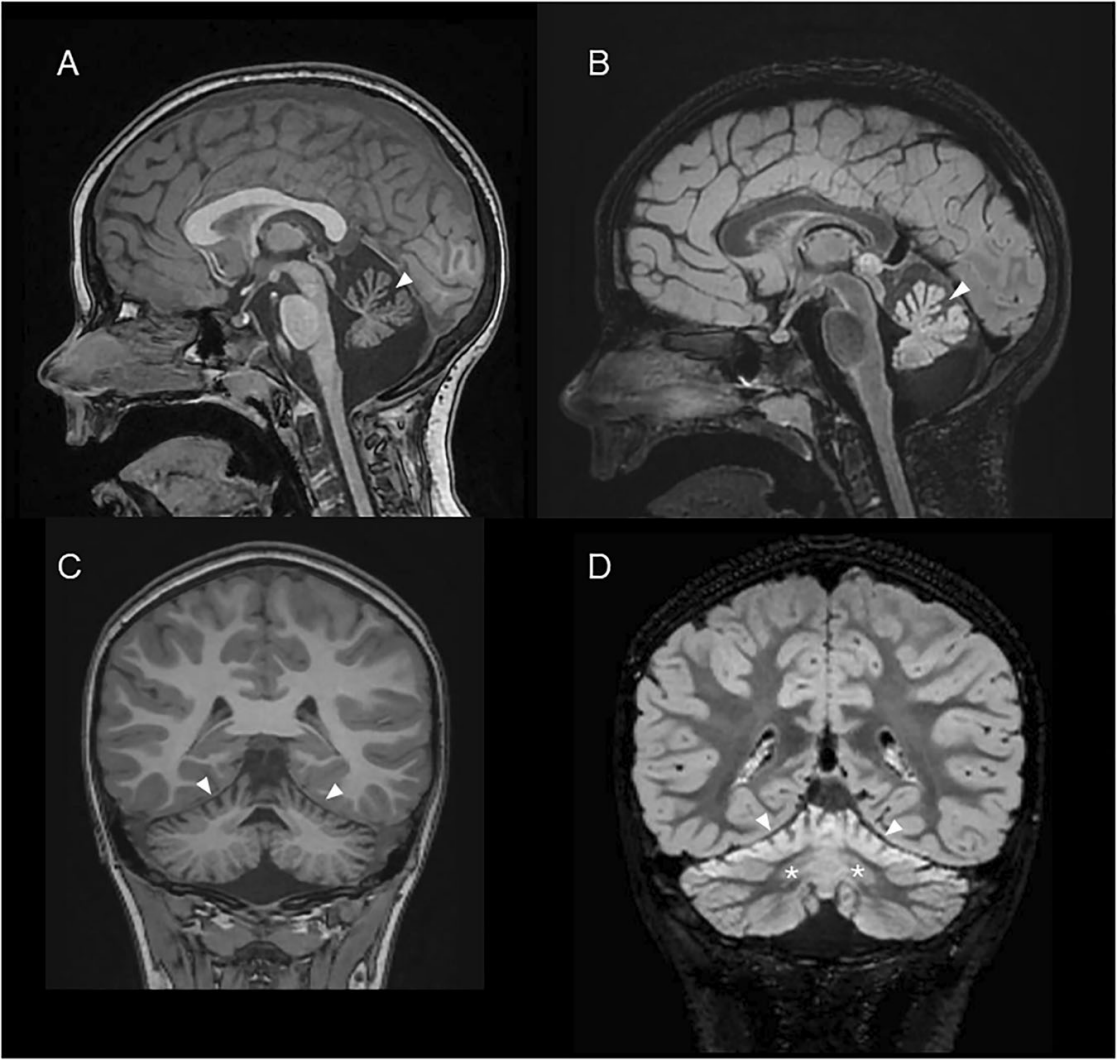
Brain MRI of MEOAL patient. Sagittal and coronal t1 MPRAGE (A, C) sagittal and coronal FLAIR3D brain images (B, D). Thinning of the superior cerebellar folia and vermis with cortical and subcortical hyperintensity of cerebellar hemisphere and vermis (arrows). Mild bilaterally hyperintensity of nucleus dentatus (*).
